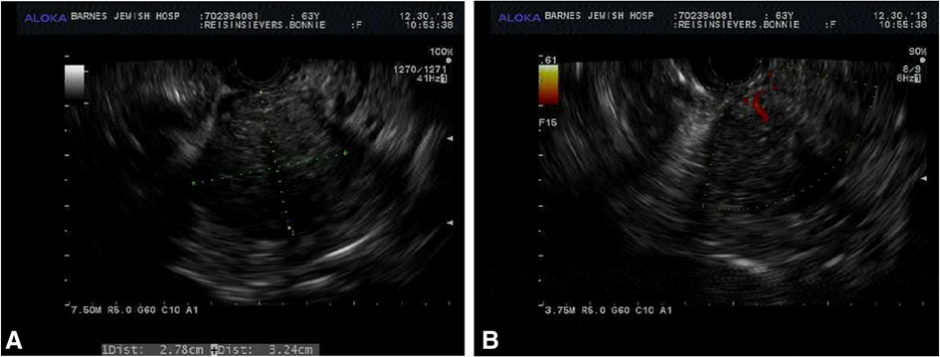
(A) Endoscopic ultrasound demonstrates a 3.2 × 2.8 cm hypoechoic discrete mass in the pancreatic body. (B) Color Doppler through endoscopic ultrasound demonstrates internal vascularity of the mass.
